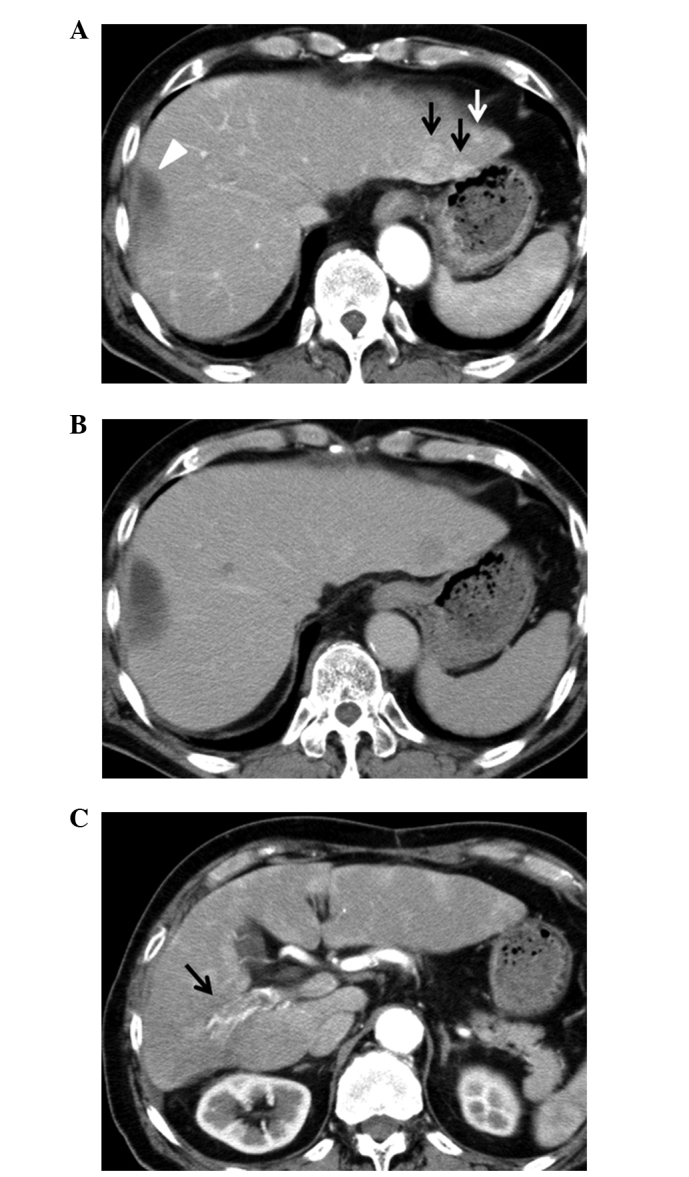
Dynamic computed tomography (CT) prior to treatment. (A) Arterial phase and (B) equilibrium phase showing several hepatocellular carcinomas (arrows) in the left hepatic lobe and biloma (arrow head) by the segmentectomy in S5 liver surface. (C) Arterial phase showing portal vein tumor thrombus (arrow) in the right portal branch.
